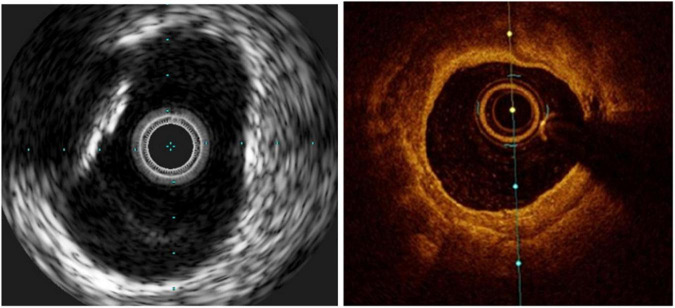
Calcific lesions at intravascular ultrasound (Left) and optical coherence tomography (Right). The limitation of IVUS in this case is that the lesion projects a shadow that does not allow measuring its depth.
